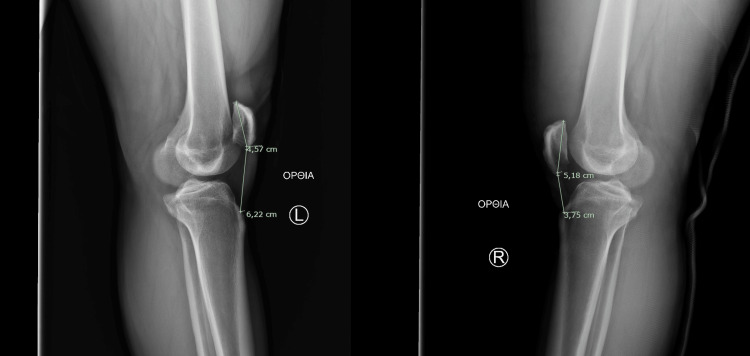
Knee X-ray with Insall-Salvati ratio Comparison between right knee (affected) and left knee (unaffected).
